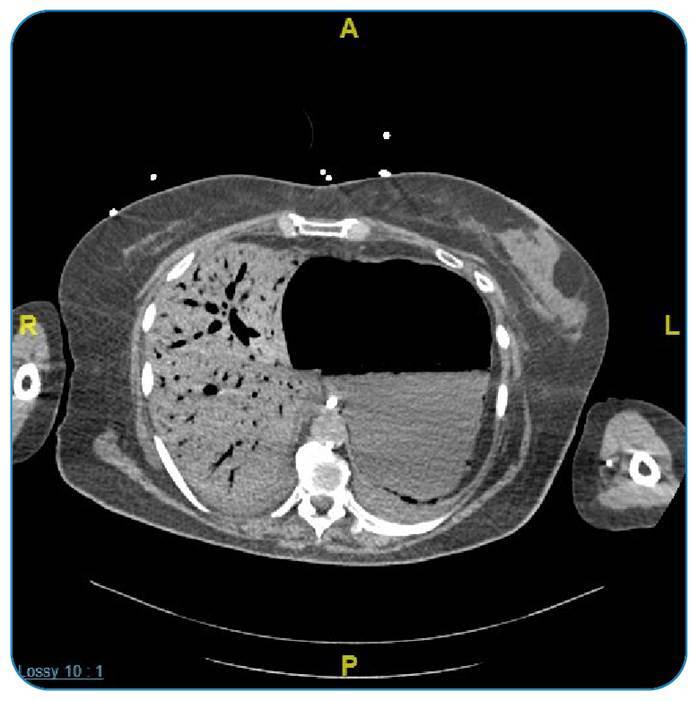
Abdominal computed tomography scan in Case 1 shows extensive portal venous gas, diffuse distension of small bowel loops, and long segments of intramural bowel gas.
